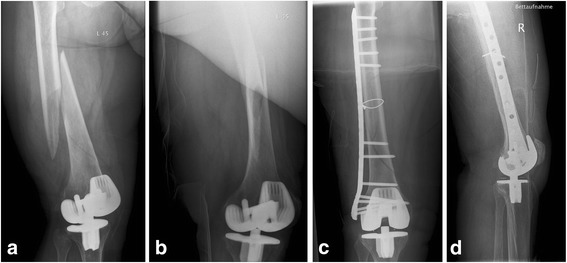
X-ray images of an 84-year-old woman with a periprosthetic fracture of the right femur with posterior stabilized (PS) TKA (type B I); a: anterior-posterior and b: lateral radiation path with representation of the fracture before supply; c: anterior-posterior and d: lateral radiation path after reconstruction and supply by plate osteosynthesis. The insertions of the distal screws were complicated by the box
